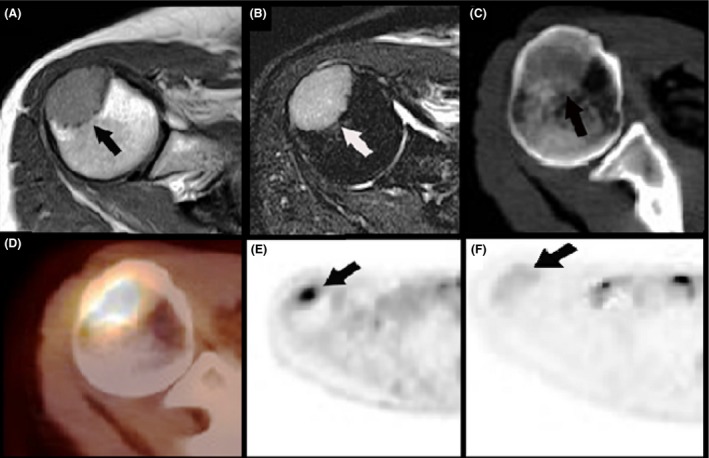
(A) Axial T1‐weighted MR image shows an intramedullary bone mass (arrow) with intermediate signal in the greater tuberosity of the proximal right humerus. (B) Axial T2‐weighted fat‐saturated MR image shows corresponding T2‐bright signal in the mass (arrow). (C) Axial unenhanced CT image shows an osteosclerotic intramedullary bone mass (arrow) in the proximal right humerus. (D) ^18^F‐FDG PET/CT fused image shows increased FDG avidity in the mass with an SUV of 7.4. (E) ^18^F‐FDG PET image shows a FDG‐avid intramedullary bone mass (arrow) in the proximal right humerus before treatment. (F) ^18^F‐FDG image following treatment 1 month later shows interval resolution of metabolic activity (arrow).
